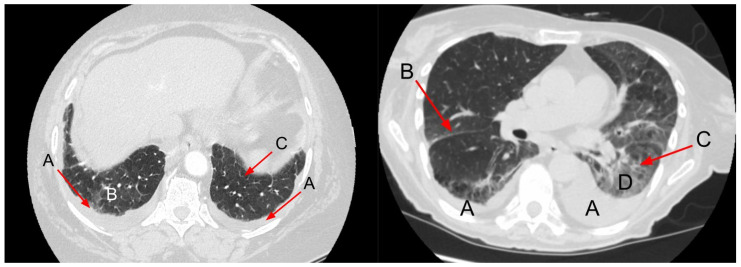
(left) Pulmonary findings related to HF in CT. A: Bilateral pleural effusion; B: Ground-glass opacities in gravity-dependent part of the lungs; C: Smooth septal thickening. (right) Image of the lungs in HF. A: Bilateral pleural effusion; B: interlobar septal thickening; C: Thickening of interlobular fissures; D: Ground-glass opacities in gravity-dependent part of the lungs. Figure obtained with permission from the 2nd Department of Radiology, Medical University of Warsaw.
